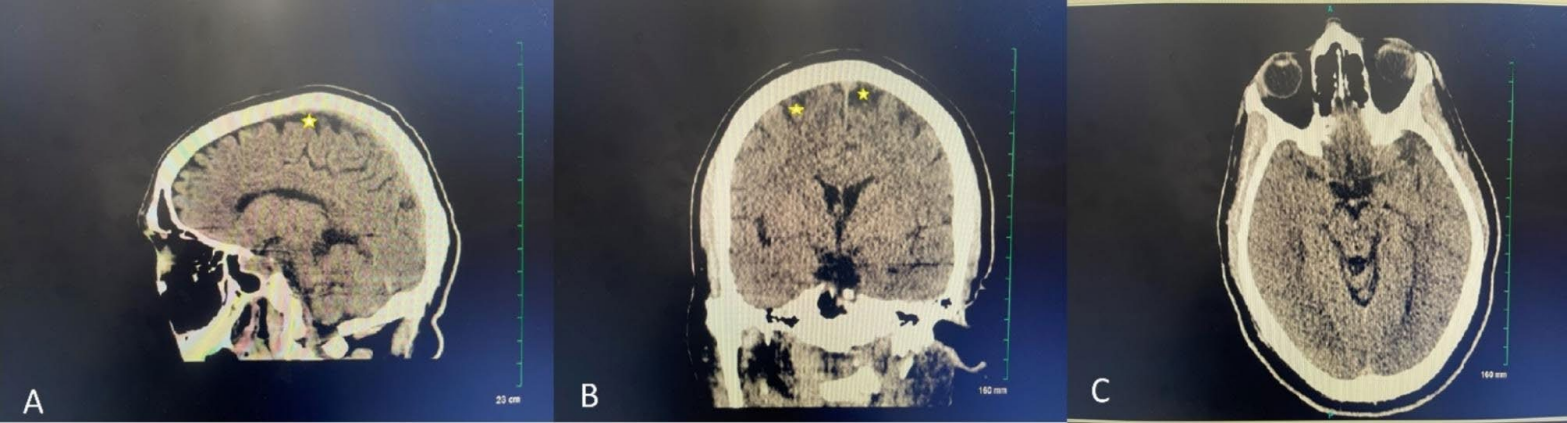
1 A Sagittal, 1B Coronal, and 1 C Axial Non-contrast CT of the brain indicating mild age-related atrophy (stars) without any acute abnormalities
